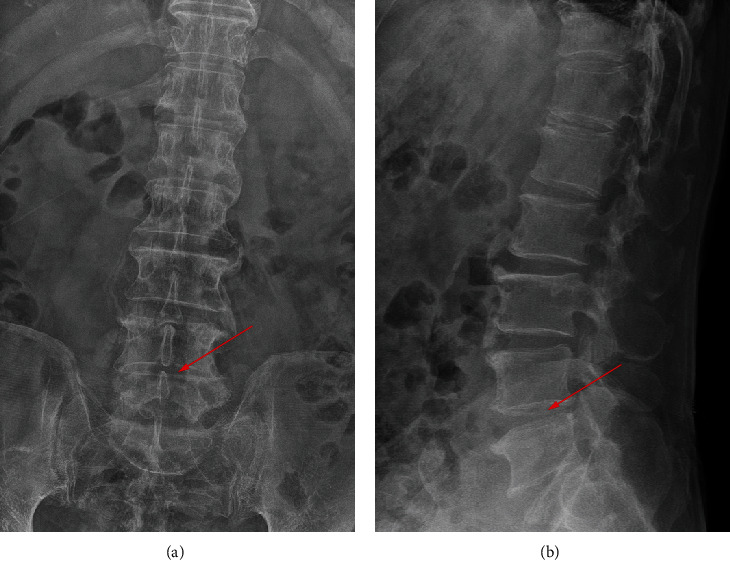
X-ray examination of a patient with nonspinal tuberculosis. The arrow points to the location of the lesion. (a) X-ray image in posterior-anterior position. (b) X-ray image in lateral position.
